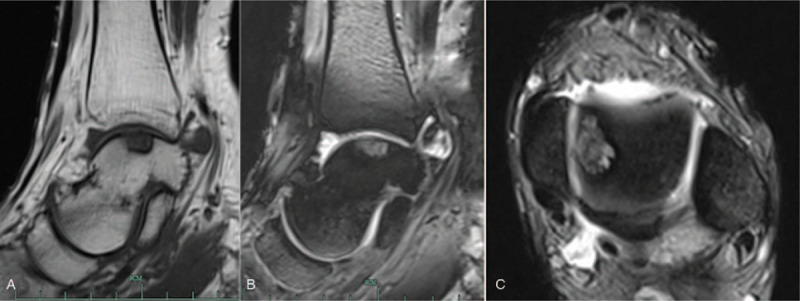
The preoperative magnetic resonance imaging (MRI) of the left ankle is shown, as well as the high signal intensity on the sagittal T1 weighted imaging, with low signal strength (A), sagittal (B), and transversal (C) T2 weighted imaging.
